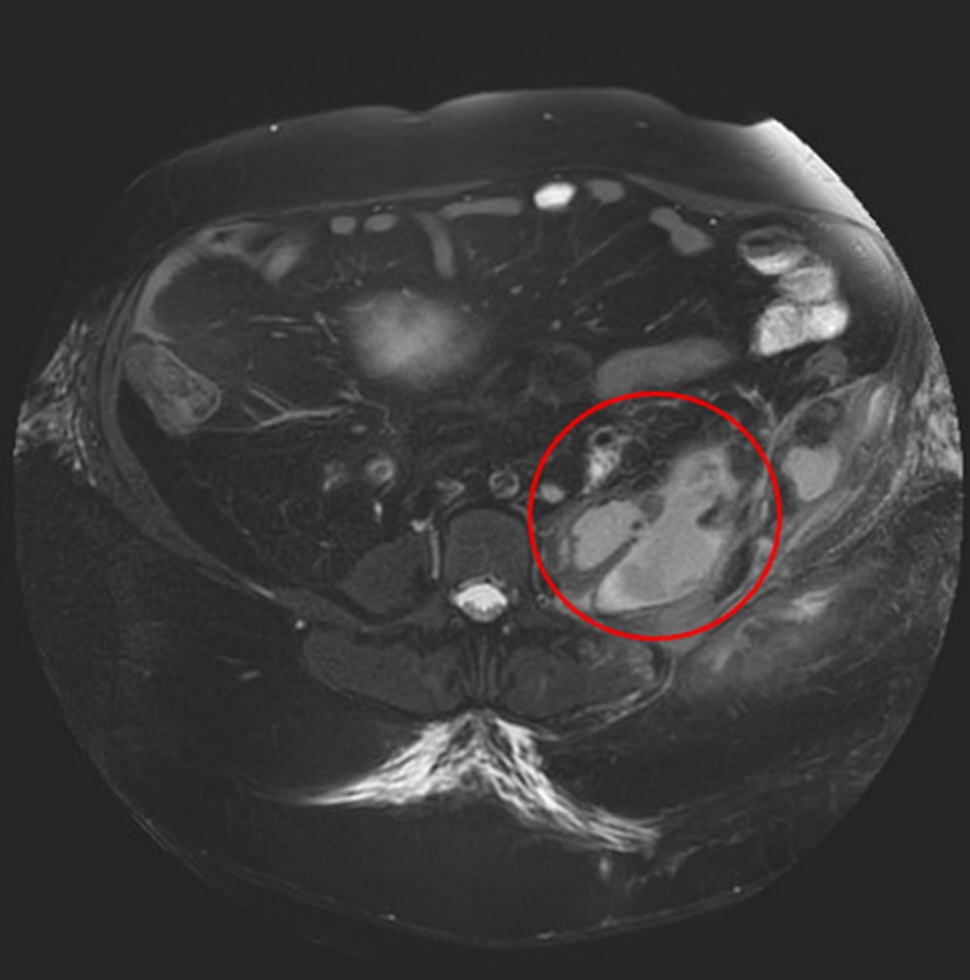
MRI in 25 + 4 weeks of gestation shows the regressed psoas abscess with a size of now 7 × 6 cm (previous: 20 × 10 cm)
